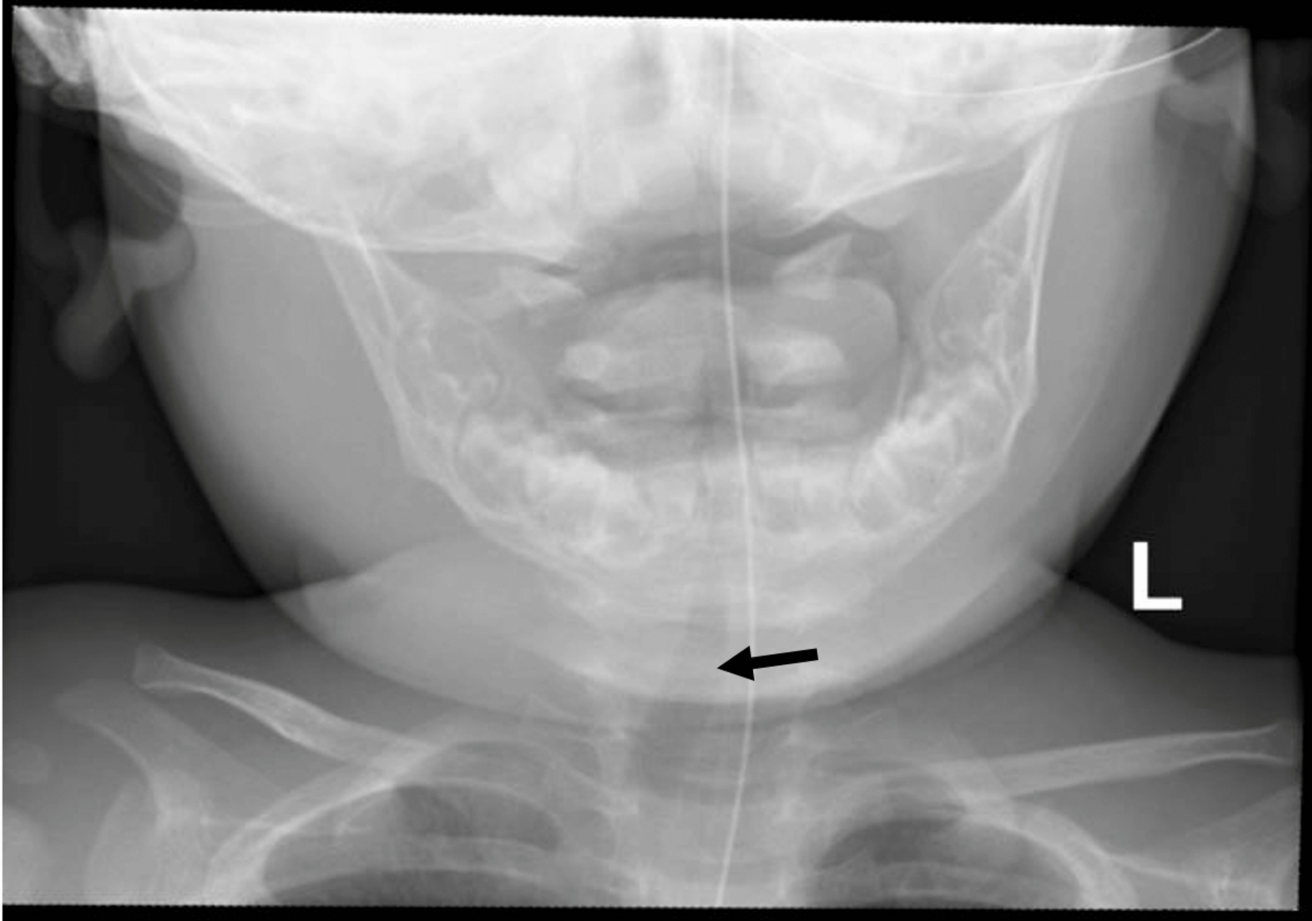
AP cervical radiograph showing the trachea (black arrow) displaced to the right. No significant narrowing is noted.
